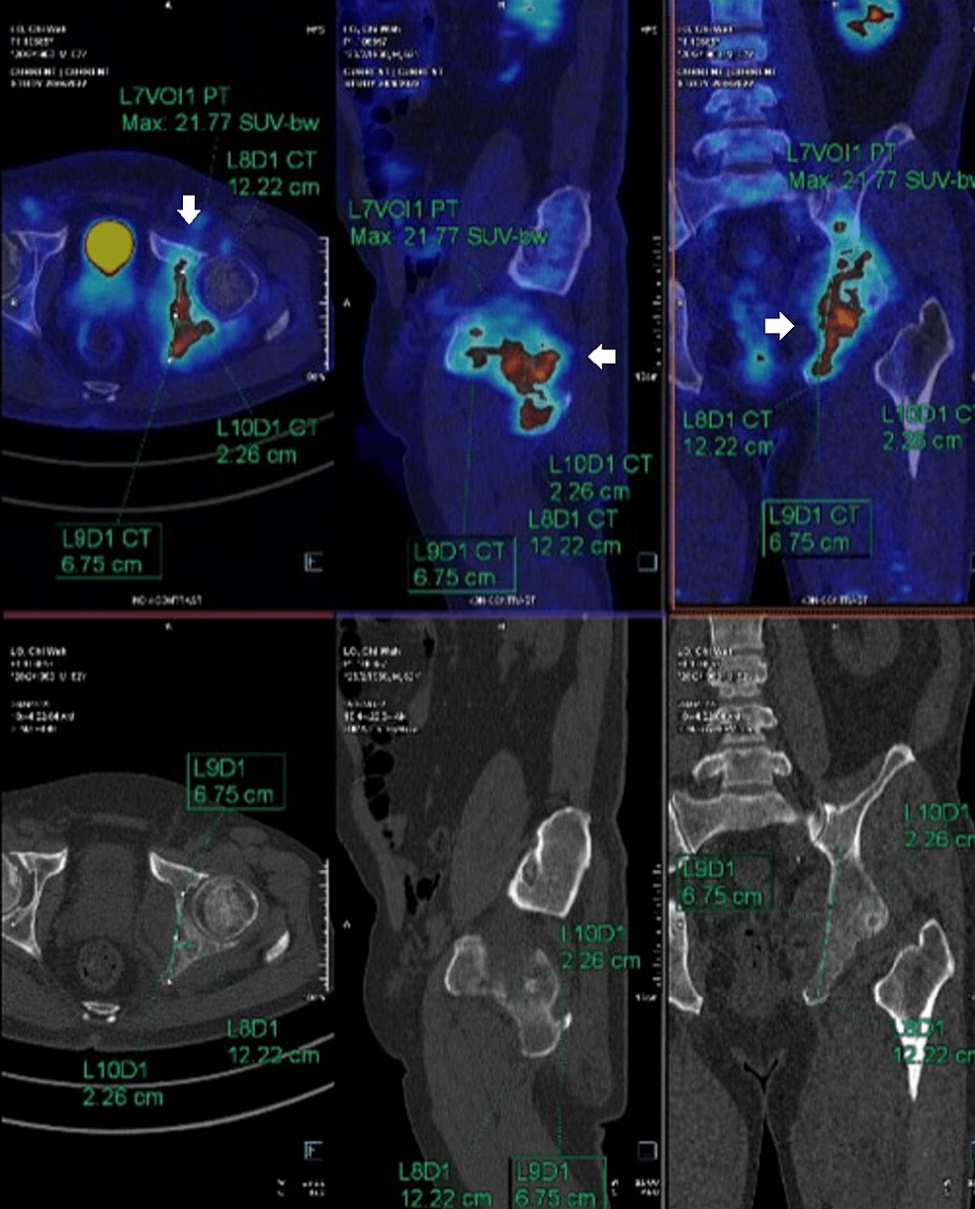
F18-PSMA whole-body PET and CT The images confirmed the carcinoma of the prostate with numerous lung and bone metastases (white arrows). More bone metastases are detected on PSMA examination. Spotty right external iliac lymph nodes, as described, represent early lymph node metastasis.
